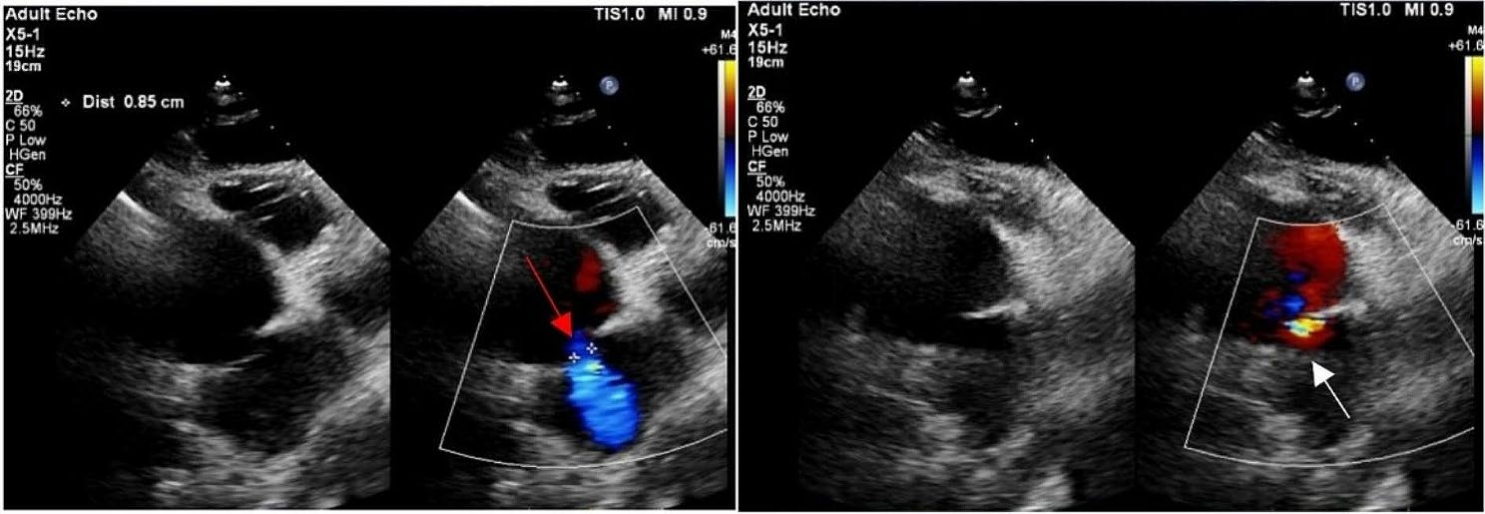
Echocardiography showing atrial septal defect and blood flow after Balloon atrial septostomy. The red arrow indicates a right to left shunt of blood flow, and the white arrow indicates a left to right shunt of blood flow
